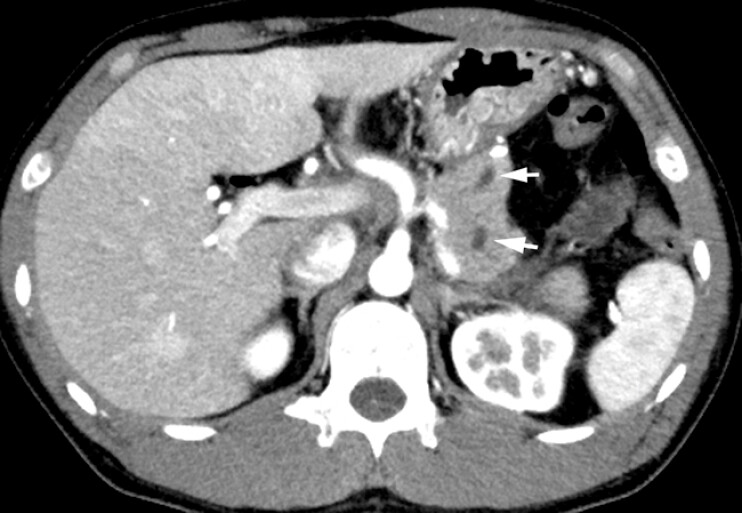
Computed tomography shows obstruction at the gastropancreatic anastomosis, as well as pancreatic duct dilatation (arrows).
